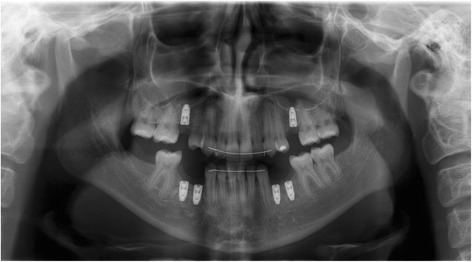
Patient 1—post-operative orthopantomogram (OPT) at age of 18
